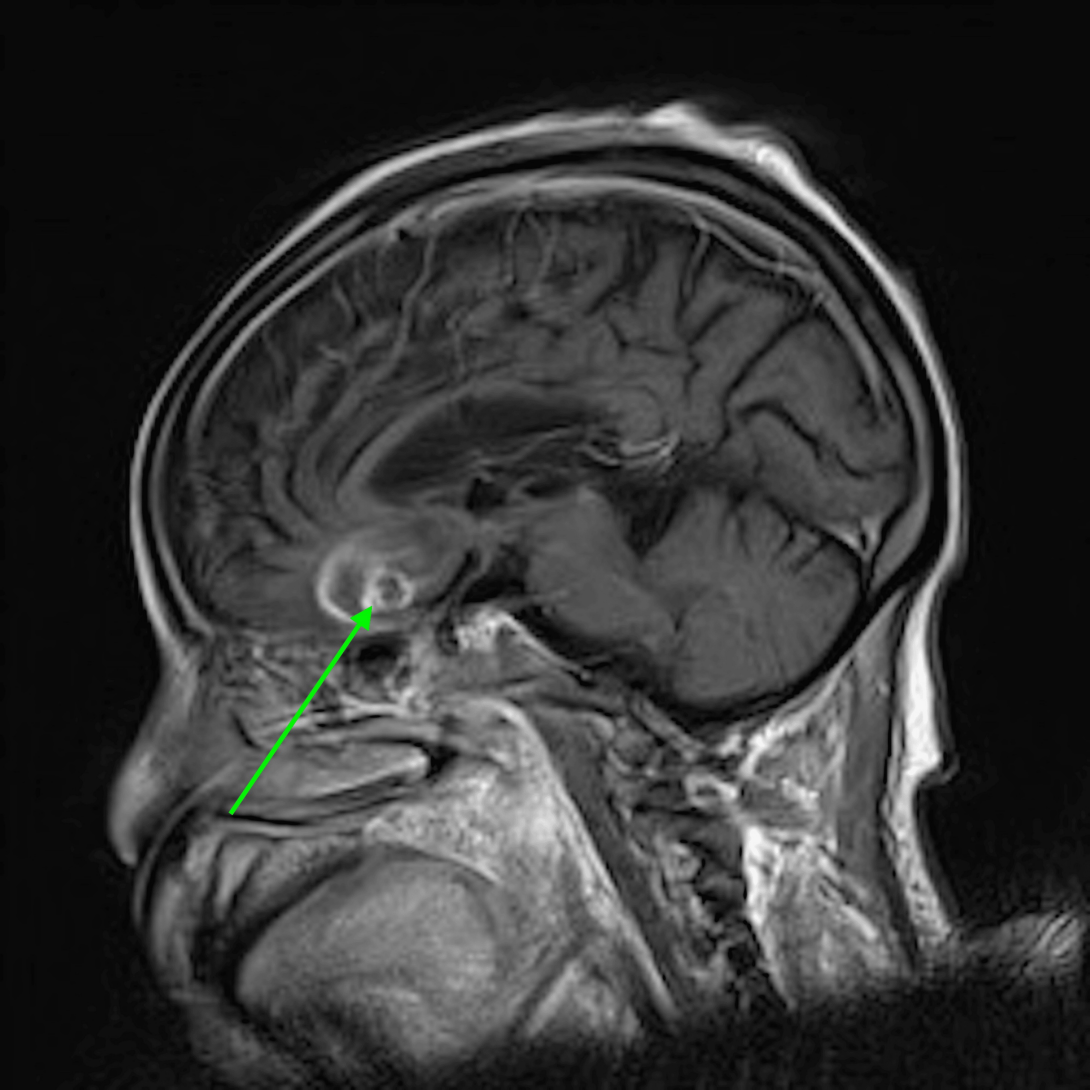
Follow-up MRI after the initiation of aggressive treatment showing multiple brain abscesses.
